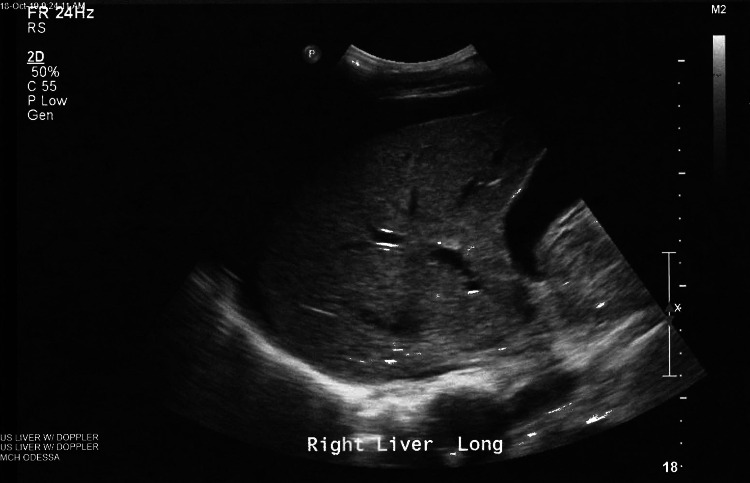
Ultrasound abdomen demonstrating normal liver echogenicity and surrounding ascitic fluid.
